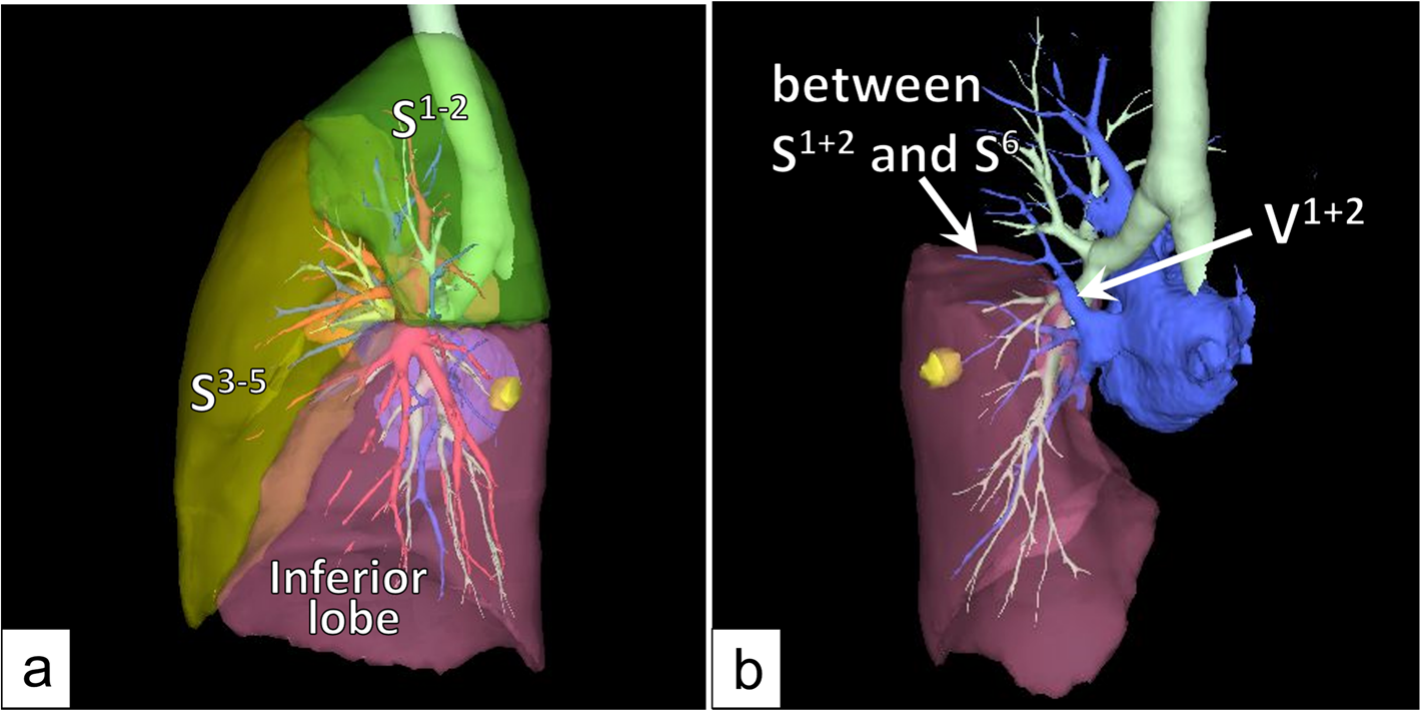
Preoperative three-dimensional chest computed tomography images. 3D-CT revealed that the volume of S^1 + 2^ was relatively large (a). The anomalous V^1 + 2^ ran from S^1 + 2^ to the left inferior pulmonary vein, and a branch of V^1 + 2^ ran between S^1 + 2^ and S^6^ (a, b).
